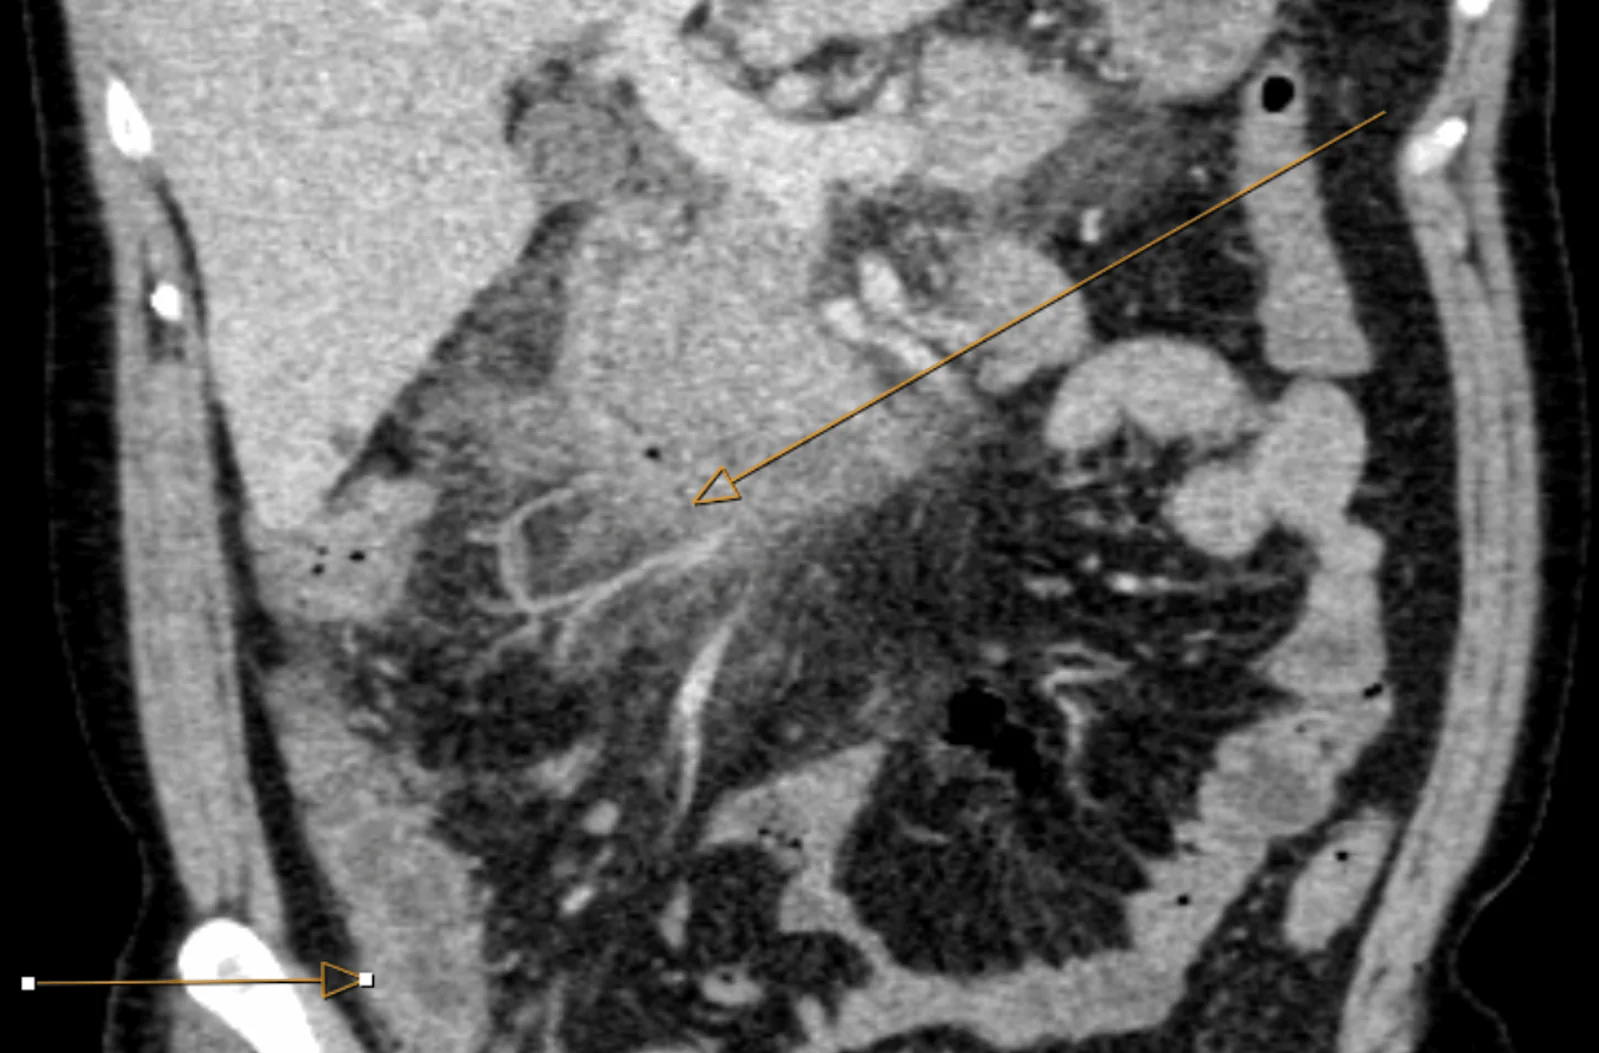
Repeat coronal contrast-enhanced CT of the abdomen and pelvis showing new wall thickening of the ascending colon, consistent with mild colitis (bottom arrow), and persistent pancreatitis (top arrow)
